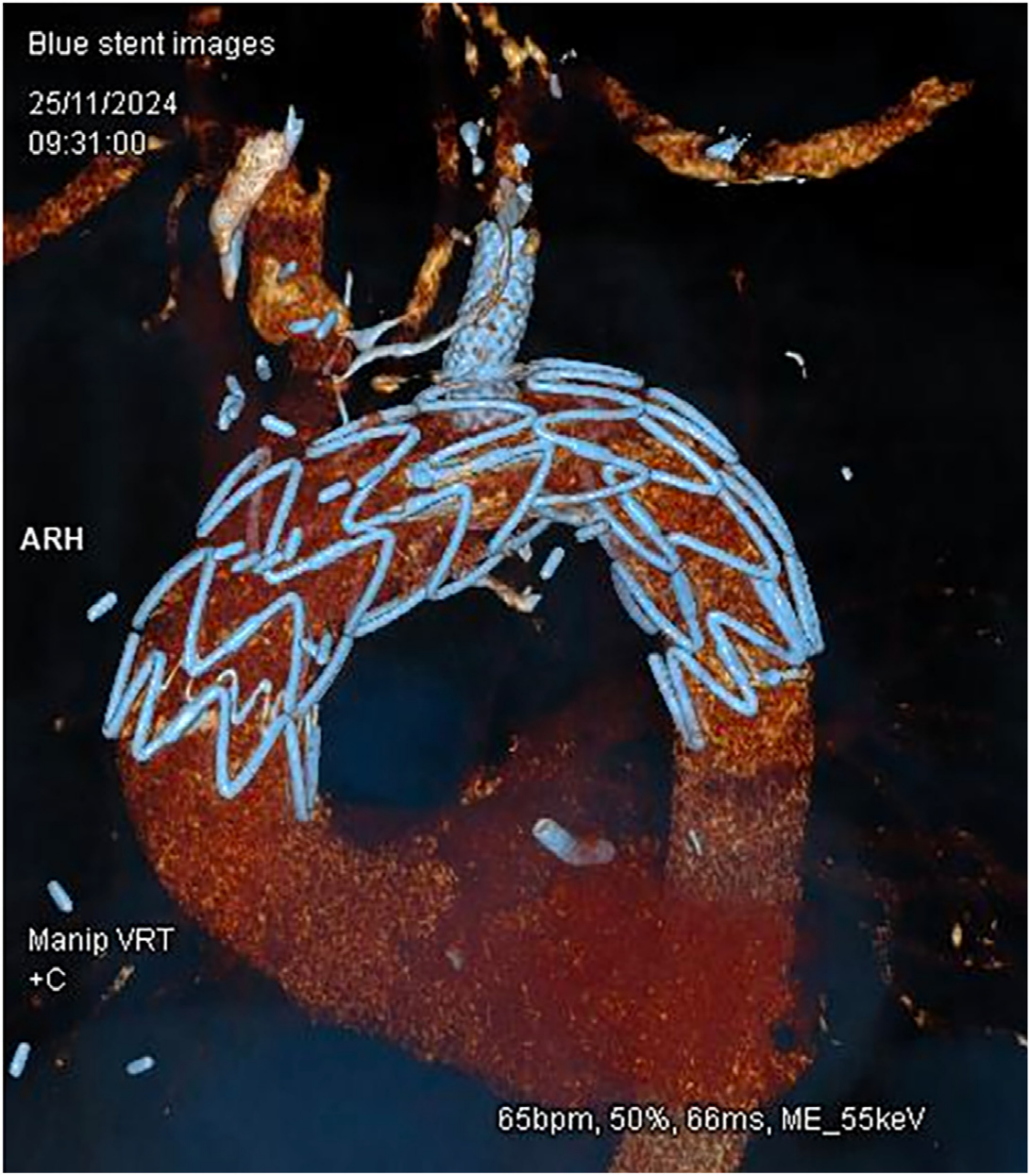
Postfenestrated thoracic endovascular aortic repair aortic arch. A follow-up 3-dimensional computed tomographic scan demonstrated that aortic graft was well deployed. Good flow to head and neck vessels with a patent stent to the left subclavian artery was noted. (ARH, aortic arch; bpm, beats/min; Manip VRT, manipulated volume rendering technique +C, contrast-enhanced.)
